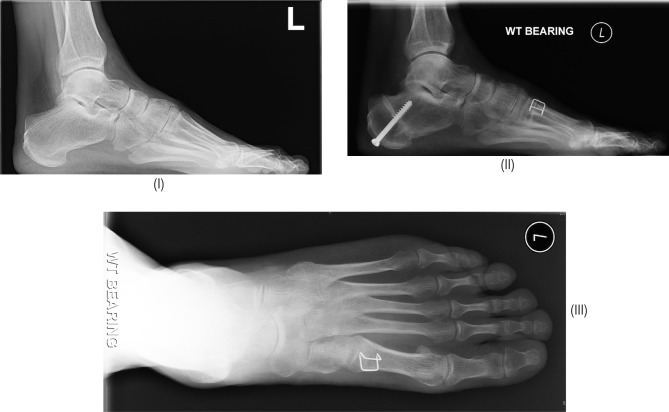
(I) Preoperative x-ray; (II) lateral weight-bearing x-ray showing healing of the basal first metatarsal osteotomy; (III) Antero-posterior weight-bearing radiograph.
